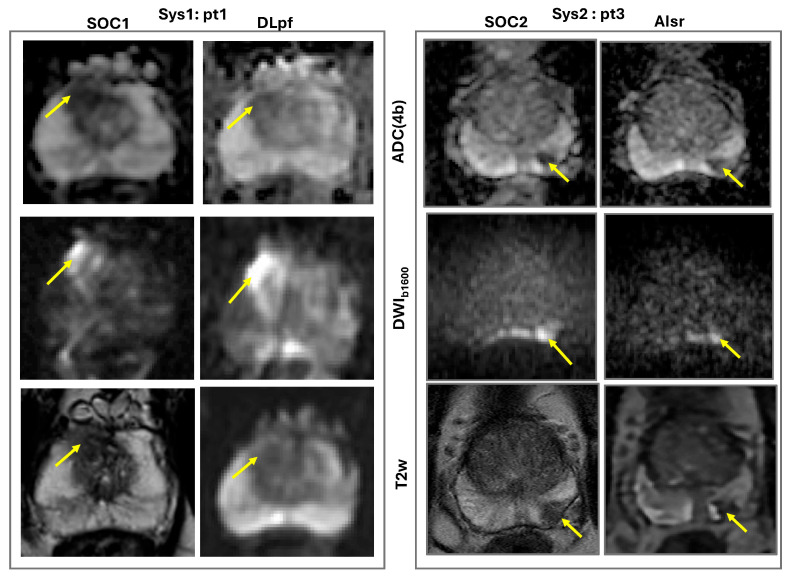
Gray-scale images qualitatively compare examples of standard of care (SOC) bi-parametric (bp)MRI versus deep-learning partial-Fourier (DLpf) and artificial-intelligence super-resolution (AIsr) from two different vendor 3T systems (Sys1 and Sys2) in two patients (pt1 and pt3) with PIRADS 4 lesions (arrows) in the right anterior mid-gland (pt1, left) and left posterior apex (pt3, right) peripheral zone.
